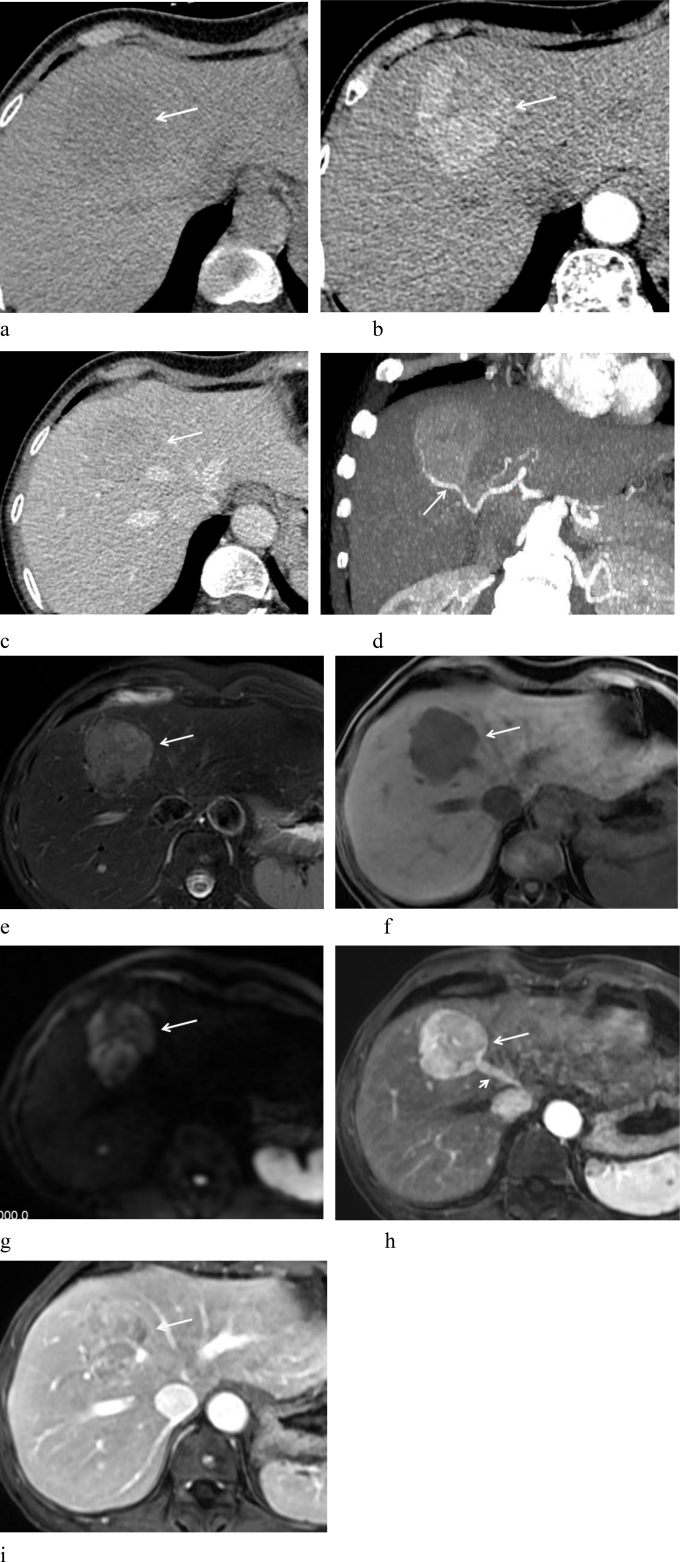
A 62-year-old woman with hepatic PEComa of malignant potential. (a) Non-contrast CT scan shows homogeneous mild hypodensity. (b) Arterial phase demonstrates marked heterogeneous enhancement. (c) Portal phase exhibits rapid contrast washout. (d) Arterial phase MIP image depicts a feeding artery (arrow) surrounding the lesion. (e) T2-weighted imaging (T2WI) reveals high signal intensity with a low-signal “pseudo-capsule” at the margin and well-defined borders. (f) T1-weighted imaging (T1WI) shows low signal intensity. (g) Diffusion-weighted imaging (DWI) displays high signal intensity. (h) Arterial phase shows prominent enhancement, with early opacification of the draining hepatic vein (short arrow), suggesting an intra-lesional arteriovenous fistula. (i) Portal phase shows rapid washout, accompanied by peripheral “pseudo-capsule” enhancement (arrow).
